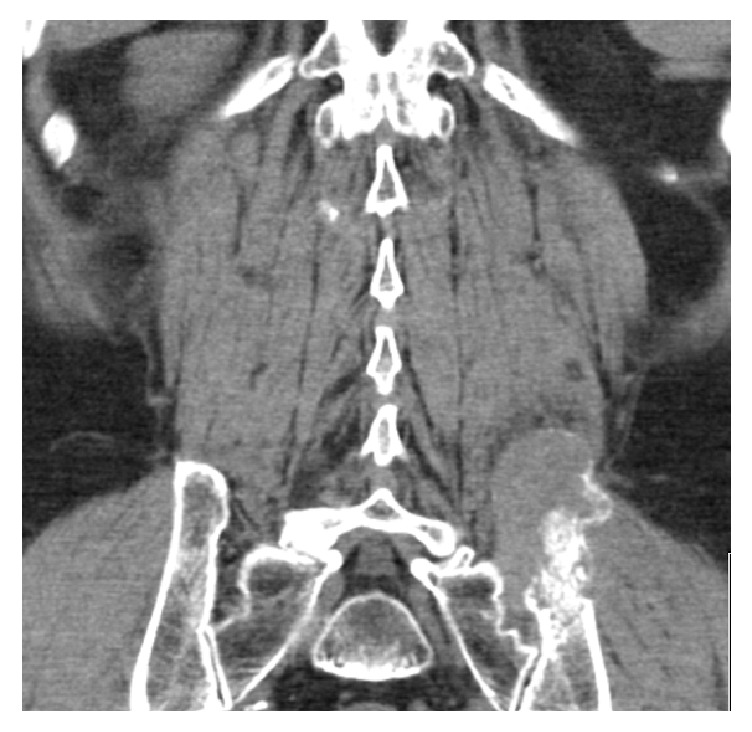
Pelvic CT revealing a 9 cm lytic lesion of left iliac bone [Case 2].
